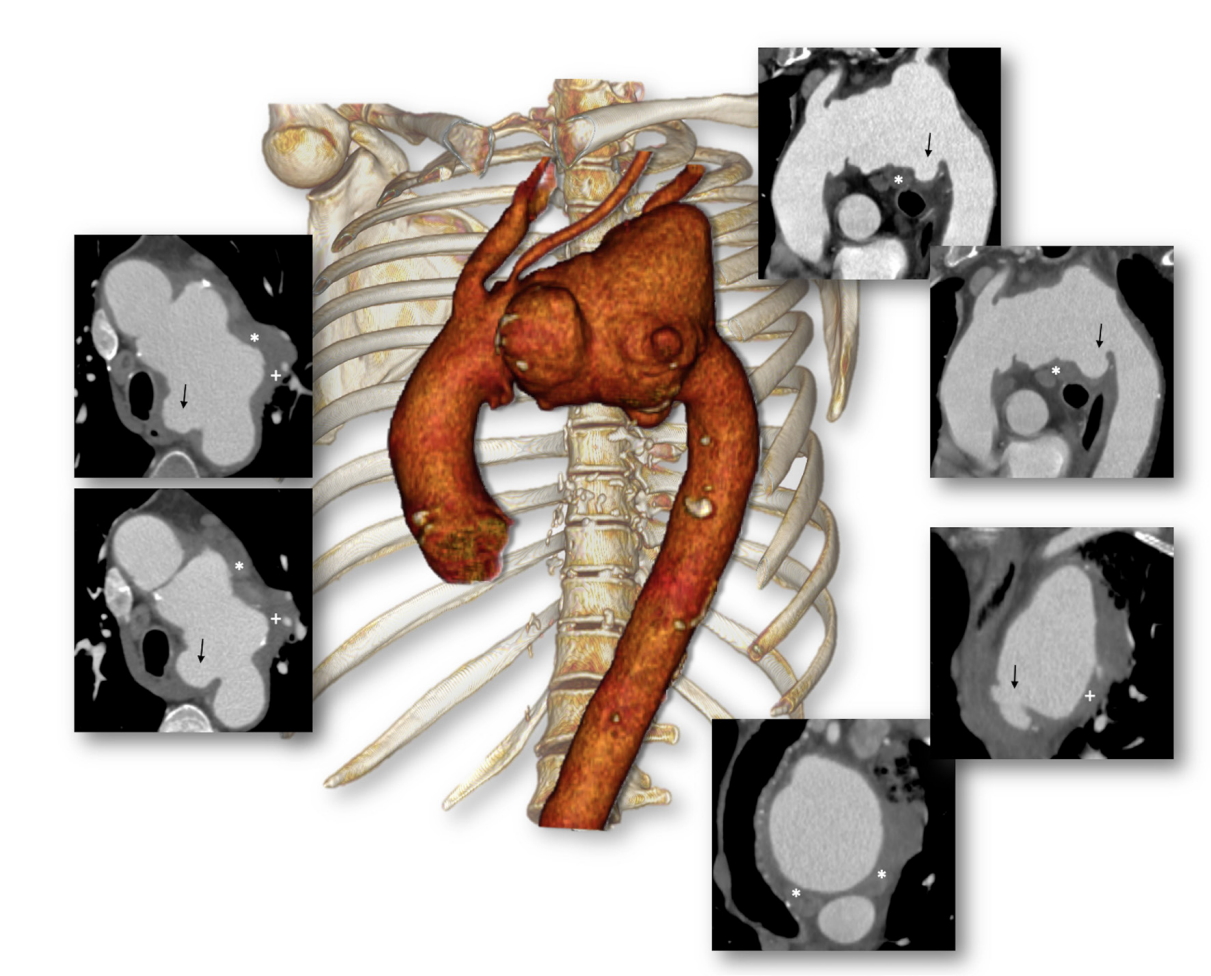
Preoperative CTA of the syphilitic aortic arch aneurysm characterized by focal and crescentic intramural hematomas (white *) - small regions of contrast accumulation with invisible or small communication with the true lumen, often with a peripheral connection with an intercostal or lumbar artery -, areas of eccentrically thickened aortic wall (white +), and ulcer-like projections with a wide neck (black arrows).
